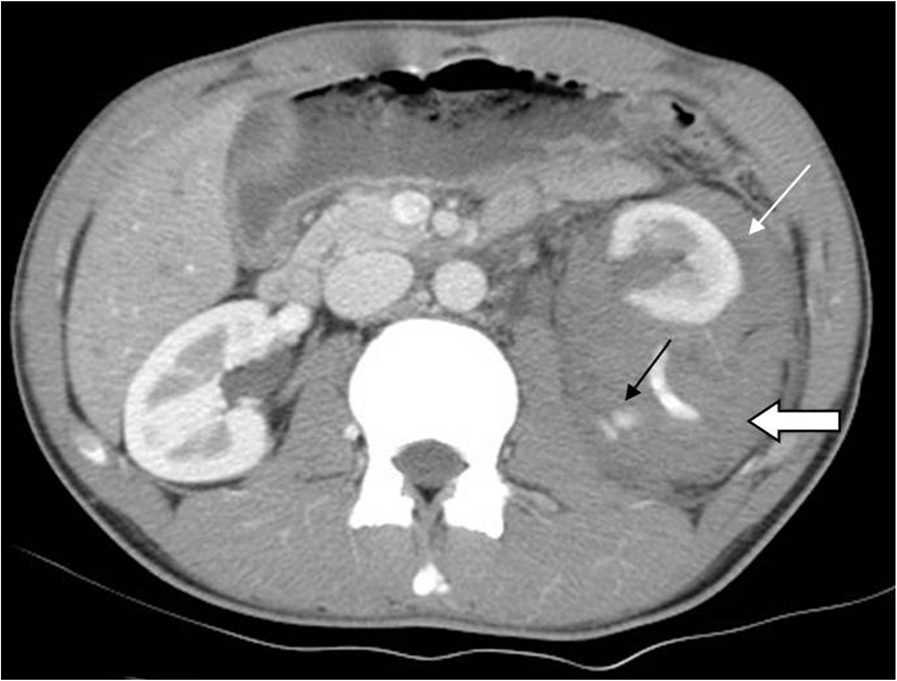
This was a 15 year-old male with grade IV left renal injury from a motor accident. Contrast enhanced CT in arterial phase in axial section showed contrast medium extravasation (*black arrow*), perirenal hematoma (*open arrow*) and pararenal hematoma (*white arrow*)
